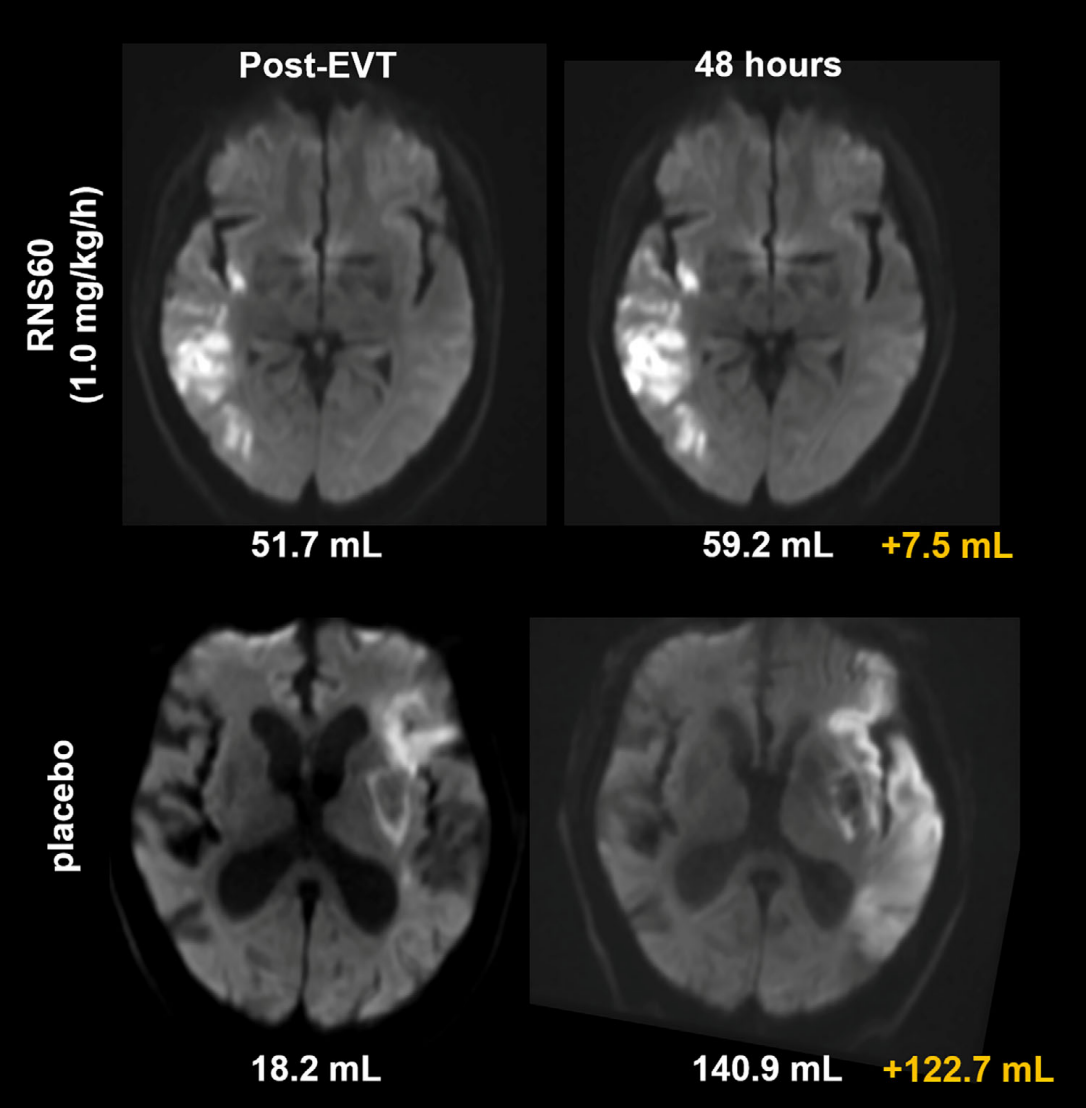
Imaging outcome of a cerebroprotective intervention started during EVT. Diffusion weighted magnetic resonance imaging (DWI) scans from two patients in the RESCUE trial. The patient receiving the verum, RNS60, at the high dose (1.0 mg/kg/h). The treatment was initiated during the EVT procedure and lasted for 48 h. The verum‐treated patient showed only marginal infarct growth in DWI whereas the patient receiving placebo treatment exhibited a dramatic infarct growth. Across the trial, patients treated with high‐dose RNS60 experienced a significantly decreased infarct growth from post‐EVT imaging to 48 h.
